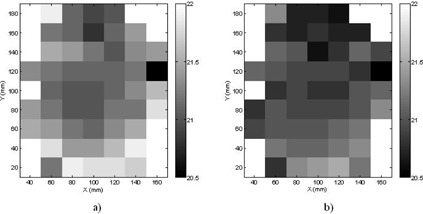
Equivalent TMR classic doses from the convolution algorithm for single 8 mm shots placed on the Z = 100 cm slice of the head CT with a prescription dose of 20 Gy: a) shots from the Perfexion; b) shots from the 4C.
